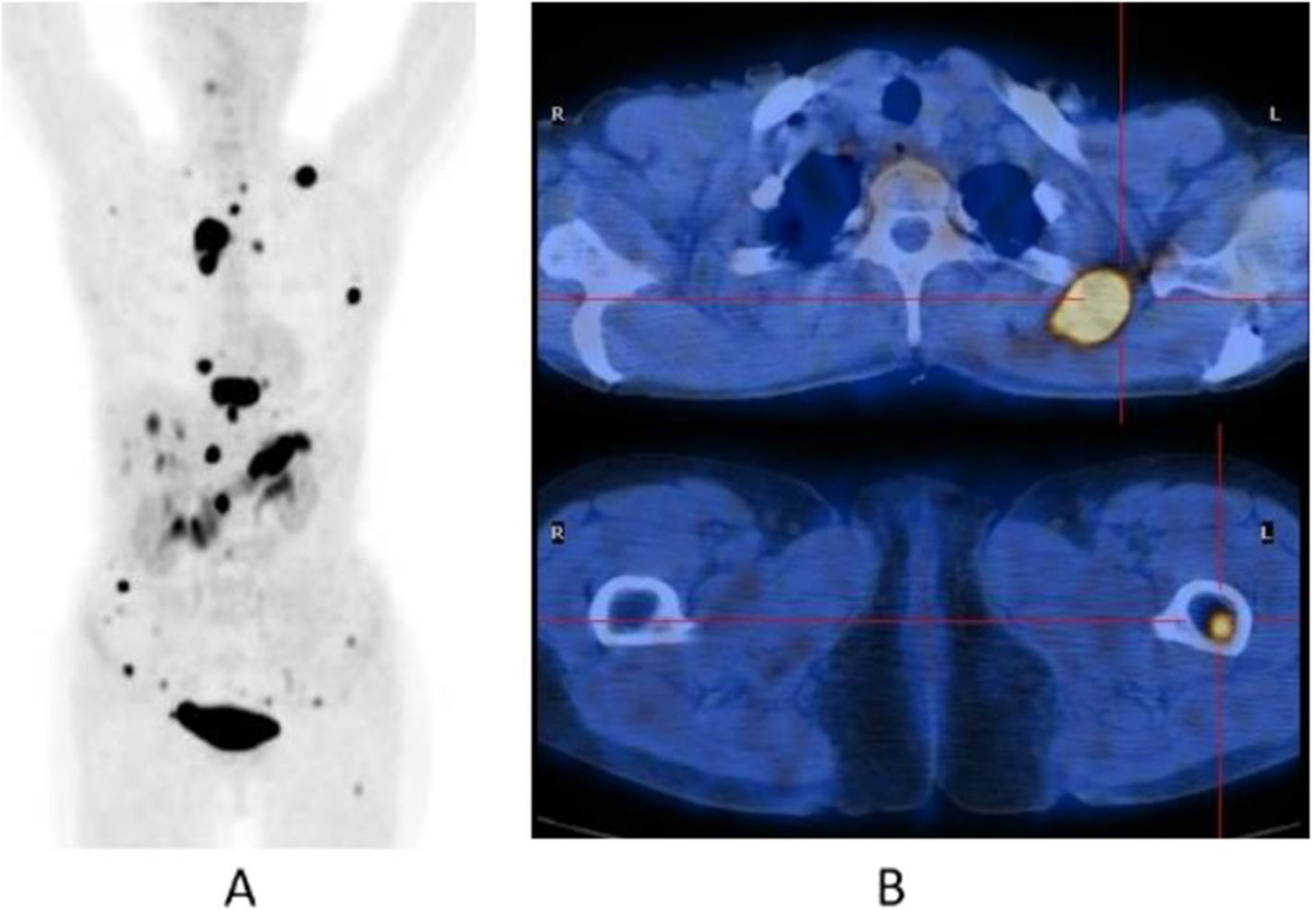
^18^F-FDOPA, carcinoid. *Clinical history*: 45 y.o. man with suspected right lung carcinoid. *PET/CT findings*: intense tracer uptake in the right lung and in multiple bone and soft tissue lesions seen on MIP (a) and fused images (b)
